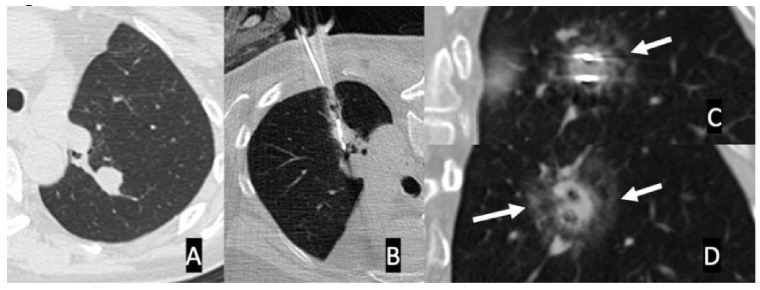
(A) Axial CT scan image demonstrating a 23 × 12 mm left lower lobe biopsy-proven adenocarcinoma. (B) Axial images with the patient prone show two antennas placed parallel with the tips extending approximately 5 mm beyond the tumor. (C) Coronal image following MWA using 30 W for 5 min shows a faint halo of ground-glass surrounding the tumor and confirming the ablation zone (arrow). (D) Following removal of the antennas, the ground-glass is better visualized and confirms adequate coverage following MWA (arrows) surrounding the tumor.
